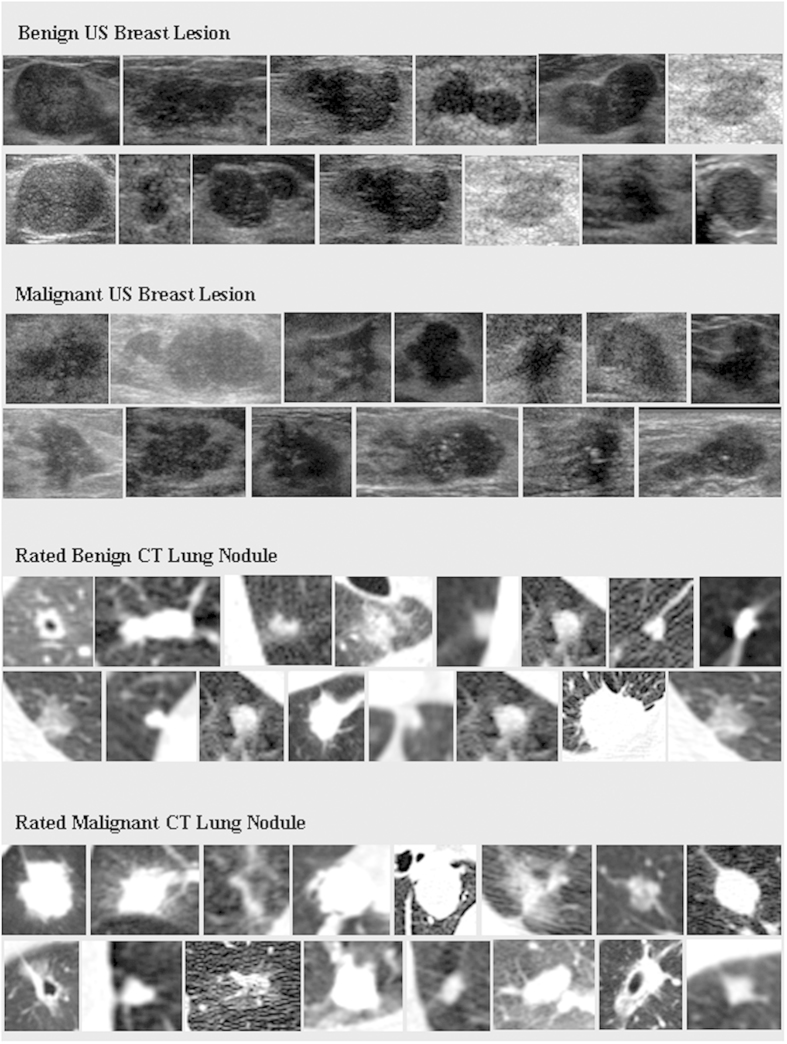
Exhibition of breast lesions and lung nodules in US and CT images.
